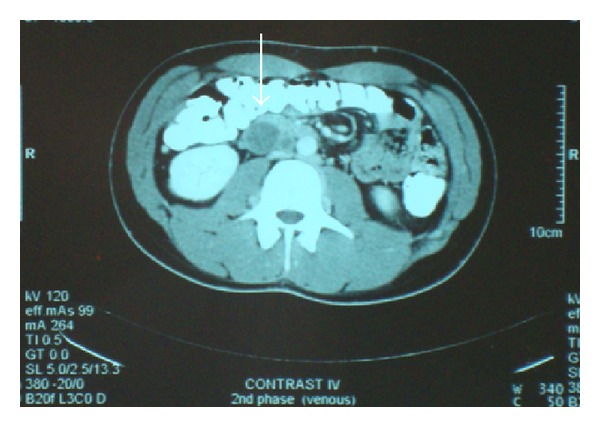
Computed tomographic angiography in patient C showing the thrombosis of the inferior vena cava along with a significant degree of perivascular inflammation.
